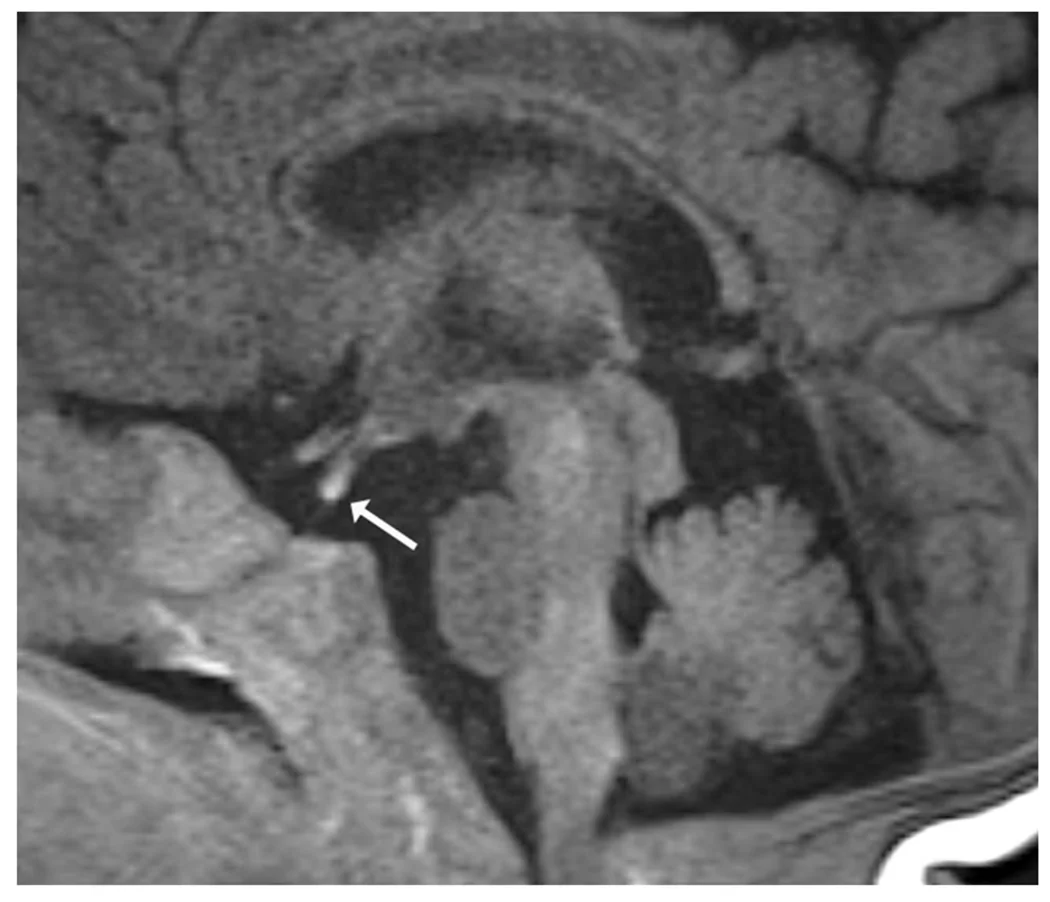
Two-month-old male infant with a history of prematurity and congenital heart disease, incidentally discovered to have ectopic posterior pituitary on brain imaging performed for neurodevelopmental prognostic reasons. Sagittal T1-weighted image focused on the region of the hypothalamus unexpectedly identifies an ectopic neurohypophysis positioned midway along the pituitary stalk between the hypothalamus and sella (*arrow*). A full panel of endocrine laboratory studies was normal at both 2 months and 4 months of age
